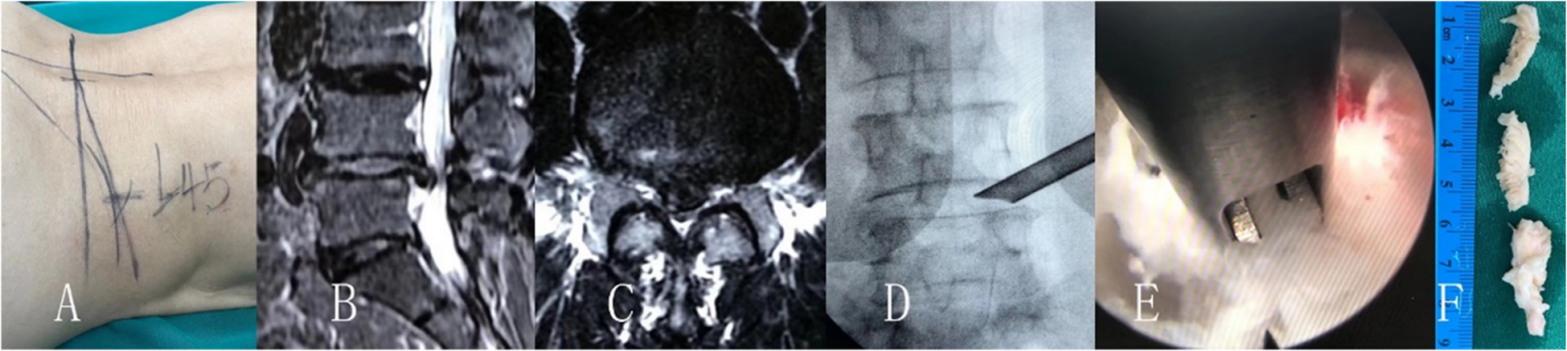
Thirty-nine years old female patient had L4/5 center disc herniation and right leg pain combine with numbness. The leg pain was disappeared after transforaminal endoscopic lumbar discectomy and nerve decompression, but the numbness was stayed until 8 months after operation. a show the skin marks, b and c was the MR image of the center disc herniation, d was the image of the endoscopic procedures, e show the herniation disc remove, and f was the removed disc herniation fragment
